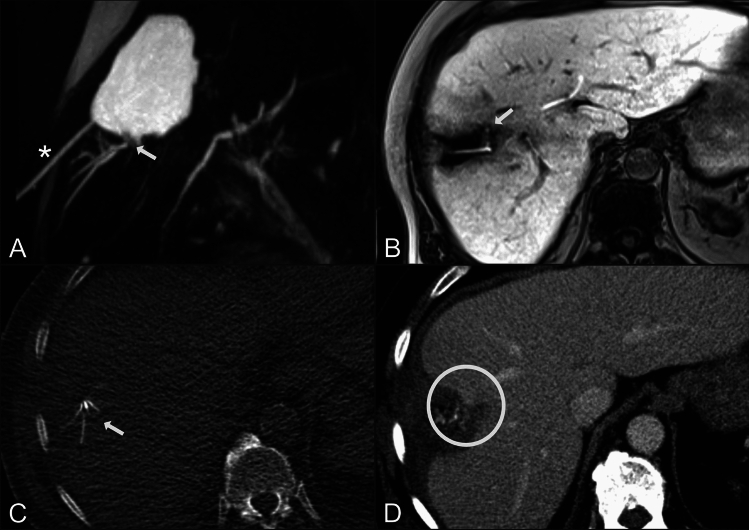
A: Pre-interventional T2w-MRCP displaying a disconnected duct (arrow) emptying into a biloma, which is already drained by a percutaneous biliary drainage (asterisk). B: Hepatocyte-specific contrast-enhanced MRI demonstrates contrast extravasation into the biloma (arrow), providing non-invasive evidence of an active bile leak. C: CT fluoroscopy demonstrates correct placement of the extended radiofrequency ablation probe (arrow) at the leakage site. D: Post-ablation contrast-enhanced CT demonstrates the ablation zone at the leakage site of the disconnected duct (circle). In this patient, the isolated bile leakage completely ceased six days after the ablation
